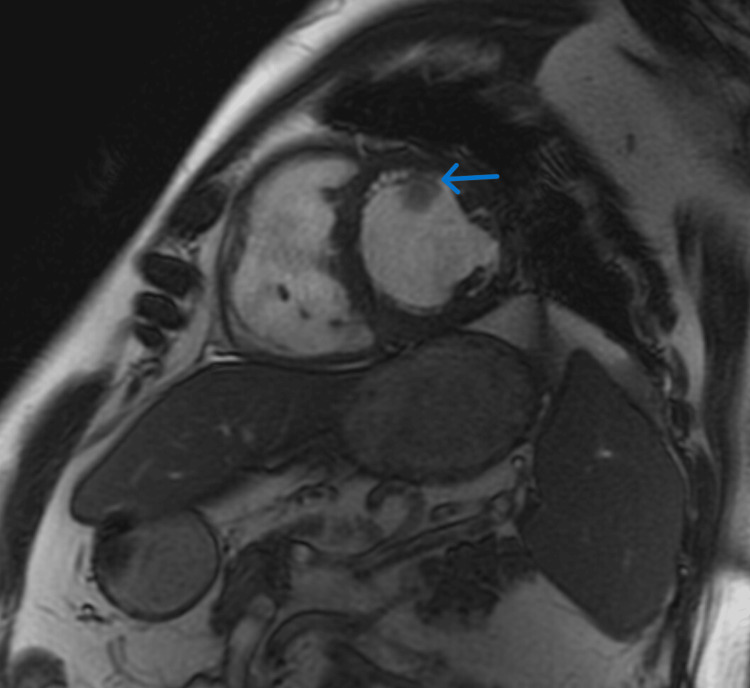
Cardiac MRI showing 17 x 17 mm mass attached to the anterior wall of the left ventricle.
